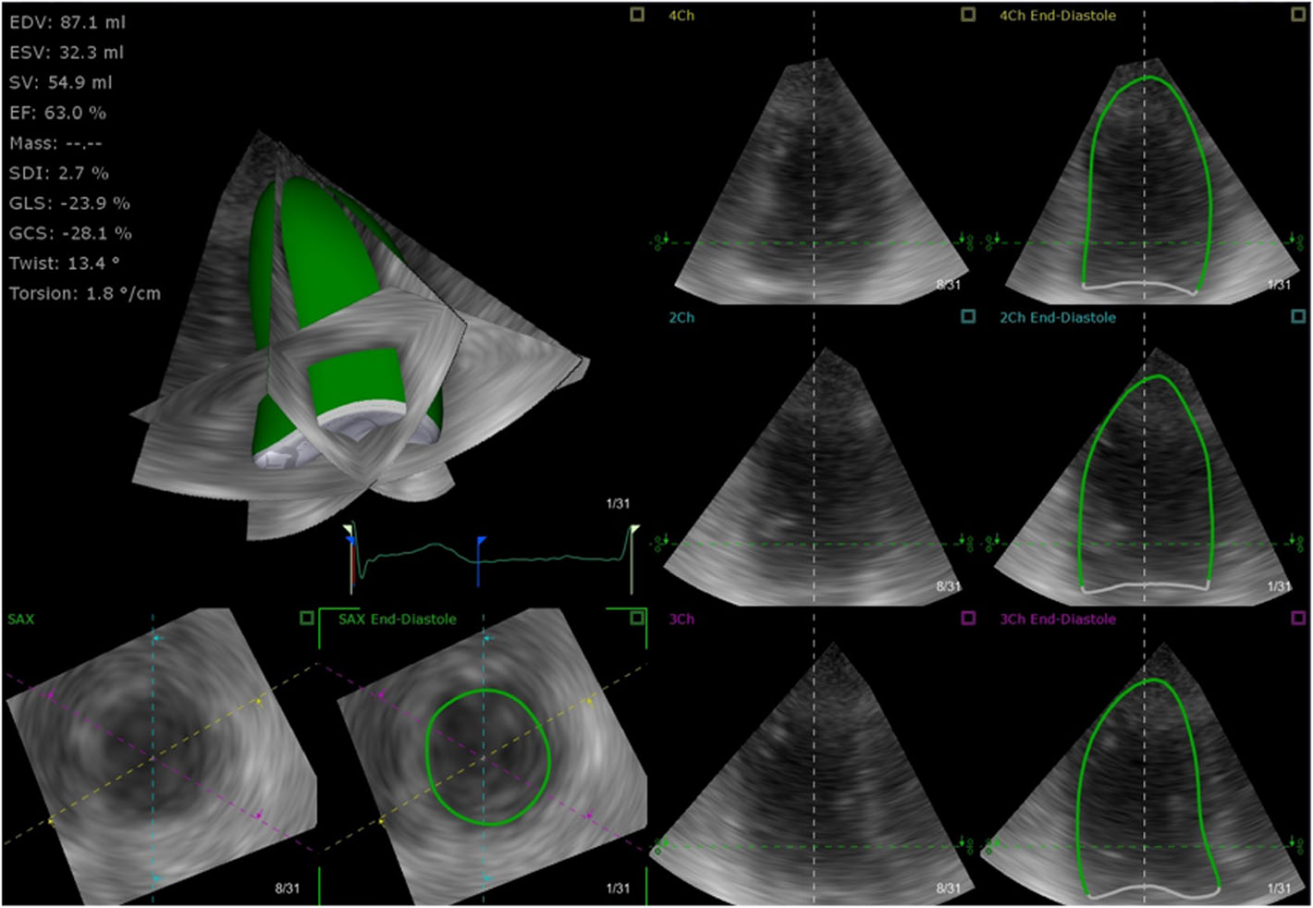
Real-time 3D left ventricular (LV) volume estimation with transthoracic echocardiography. The 3D LV volume is displayed (opaque green structure) with ‘cut planes’ provided in the short axis as well as four-, two- and three-chamber views. Semi-automated software estimates the endocardial border (*green line*) and its movement throughout the cardiac cycle. The user can alter this at end-systole and end-diastole if needed. *2Ch* two-chamber view, *3Ch* three-chamber view, *4Ch* four-chamber view, *EDV* End-diastolic volume, *EF* ejection fraction, *ESV* end-systolic volume, *GCS* global circumferential strain, *GLS* global longitudinal strain, *SAX* short-axis view, *SDI* systolic dyssynchrony index, *SV* stroke volume
